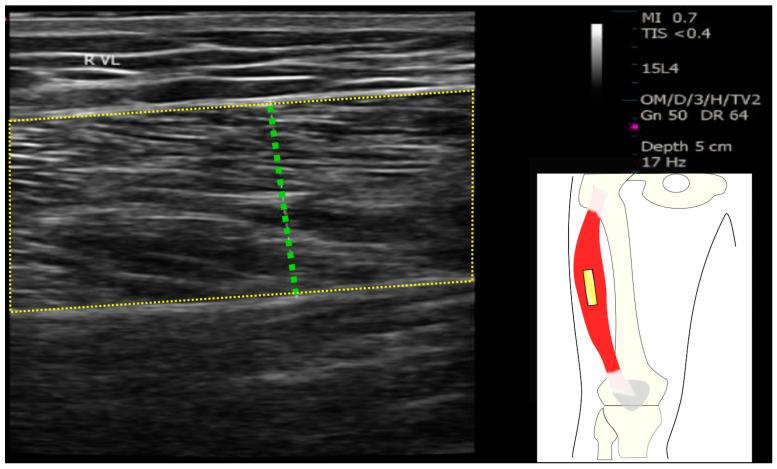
Ultrasound measurement and transducer placement on the vastus lateralis. The muscle thickness of the vastus lateralis was depicted by the green dotted line, while the echo intensity was obtained from the yellow region of interest.
